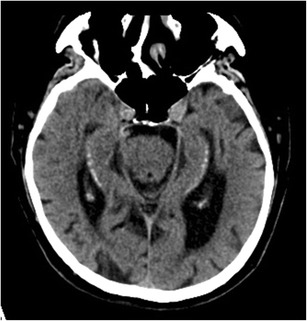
Hippocampal calcifications. Non-enhanced axial CT images angulated parallel to the hippocampal body show bilateral symmetrical calcifications lateral in the hippocampal bodies as a coincidental finding in a 69-year-old female patient with dysarthria
